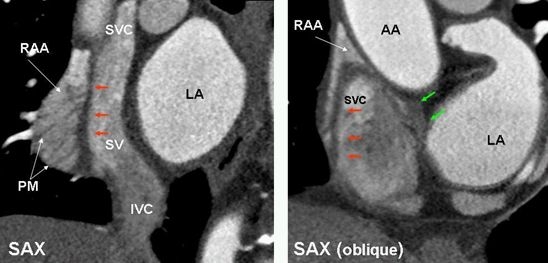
Short axis images in two different patients demonstrate the crista terminalis (red arrows) as a dark band between right atrial appendage (RAA) and sinus venarum (SV) extending from the superior vena cava (SVC) to the inferior vena cava (IVC). Superiorly, the CT arches anterior to the orifice of the SVC and extends to the area of the anterior interatrial groove and merges with the interatrial bundle, commonly known as Bachmann's bundle (green arrows). Prominent pectinate muscles (PM) are seen.  LA=left atrium, AA=ascending aorta.
